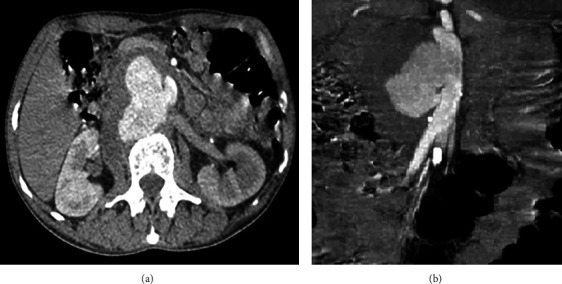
CT scan images showing the aortic pseudoaneurysm in an axial (a) and sagittal (b) view.
